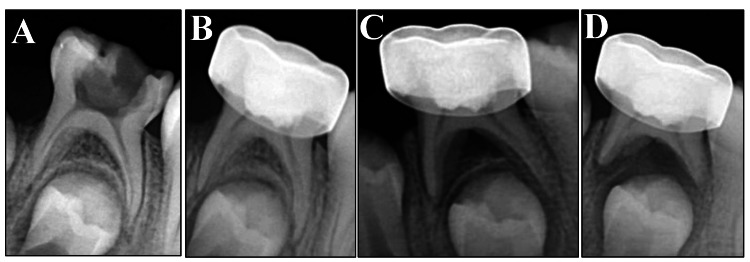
Periapical radiographs of a failed case. (A) Periapical radiograph of a primary molar showing extensive occlusal caries. (B) Immediate postoperative radiograph after the pulpotomy procedure. (C) Periapical bone and root resorption at the six-month follow-up. (D) 12-month follow-up periapical radiograph showing continued bone and root resorption involving all the primary tooth roots and the bifurcation area.
